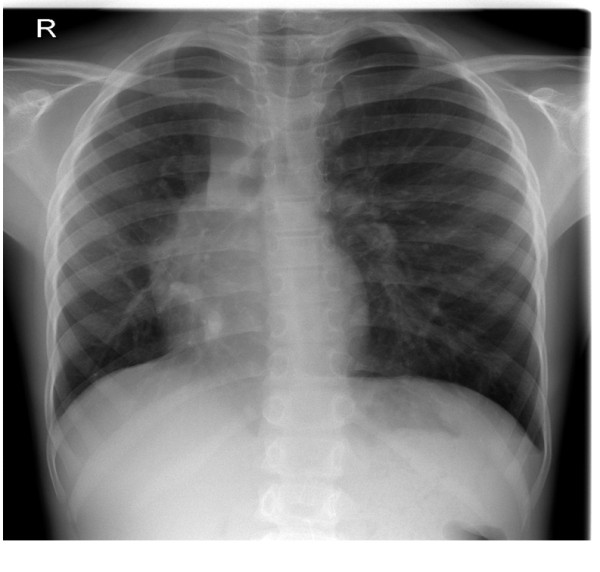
Preoperative Chest X-ray showed a dextroposition and mesocardia with scimitar vein.
